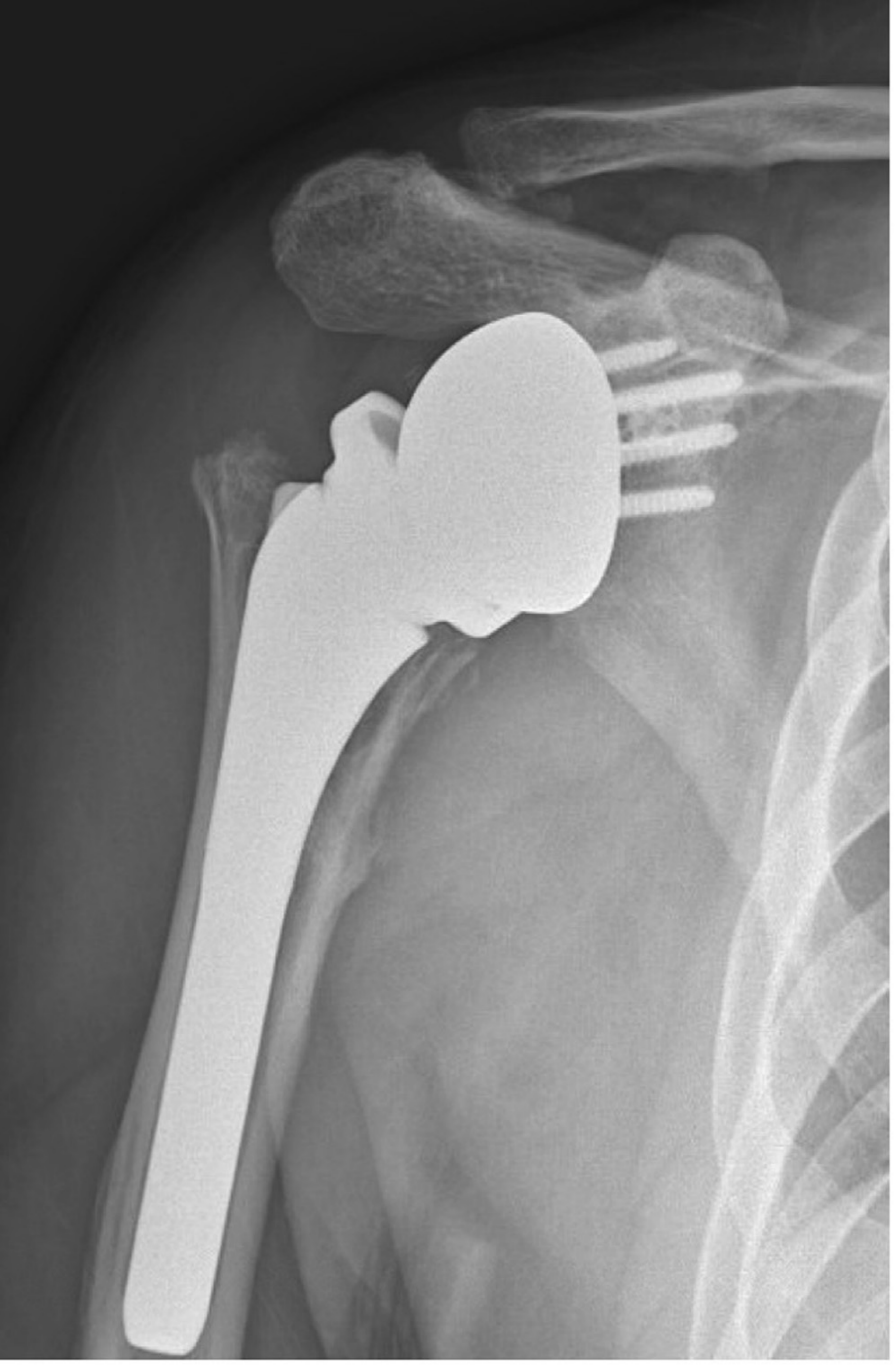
AP radiograph of the right shoulder demonstrating PDH, which should be considered a contraindication to closed reduction of RTSA. *AP*, anteroposterior; *PDH,* polyethylene dissociation from humeral tray; *RTSA,* reverse total shoulder arthroplasty *∗P<*.05.
